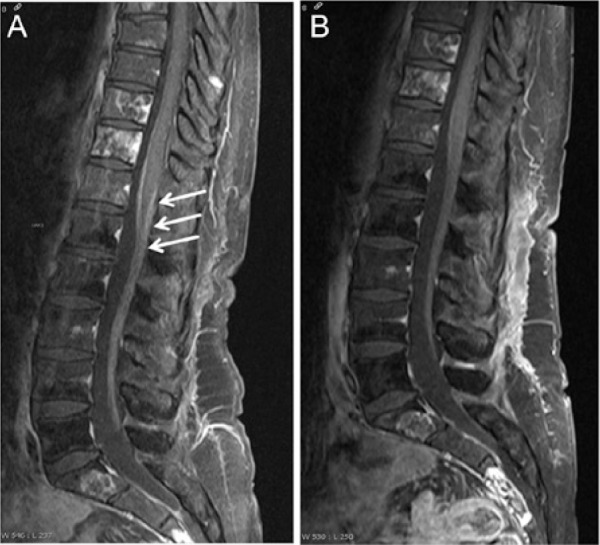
(A) MRI long spine T1 weighted with contrast medium (August 2013): enhancement of Conus medullaris and fibers of the Cauda equina (arrows), disseminated metastases of vertebral bodies. (B) MRI long spine after treatment (September 2013): T1 weighted with contrast agent: the former enhancement of Conus medullaris and Cauda equina can only minimally be seen any longer.
